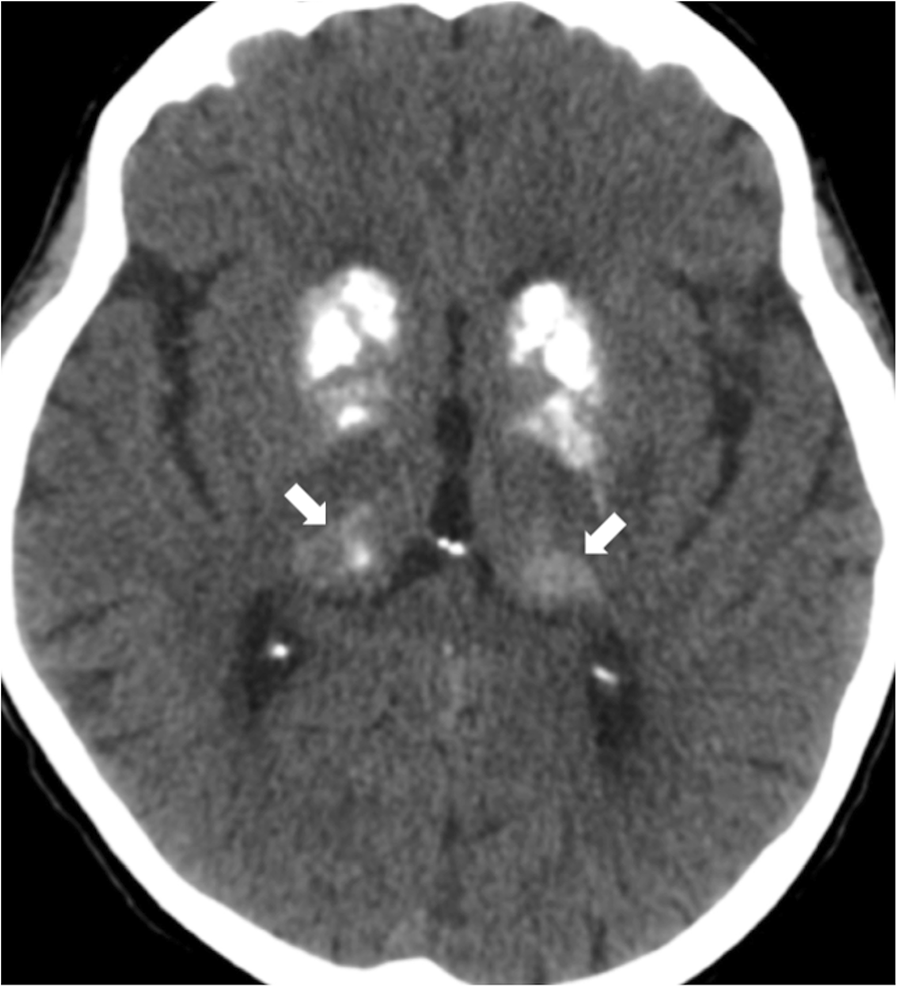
High attenuation in the basal ganglia and thalami (arrows) on non-contrast CT due to calcifications in a patient with Fahr’s disease
